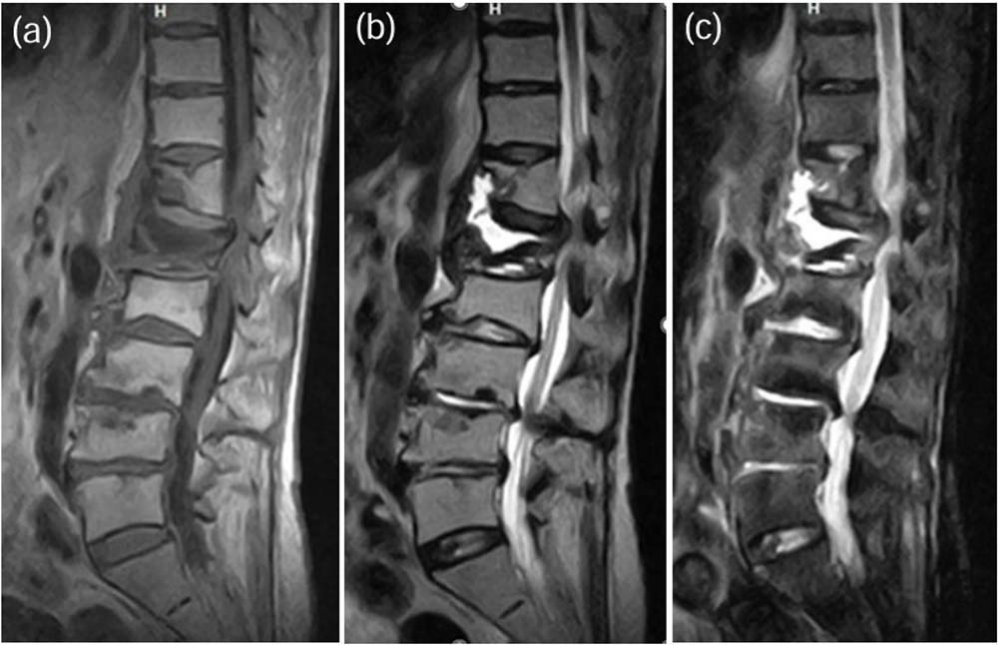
MRI demonstrated fluid signals in the L1 vertebral body and the anterior paravertebral area at Th12-L1. These were low-intensity on T1-weighted images (a) and high-intensity on T2-weighted images (b) and not suppressed on STIR images (c).
